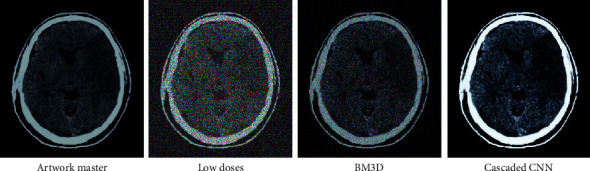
Comparison of CT images of the brain.
